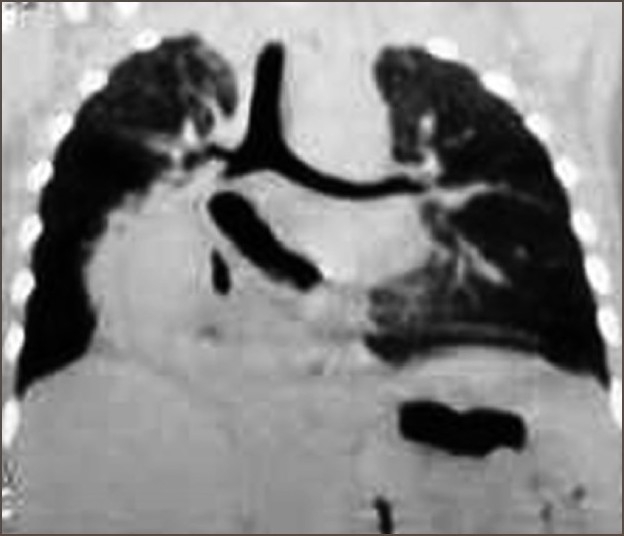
Coronal reconstruction of CT scan showing contrast filled stomach in the posterior mediastinum on right side of the chest
